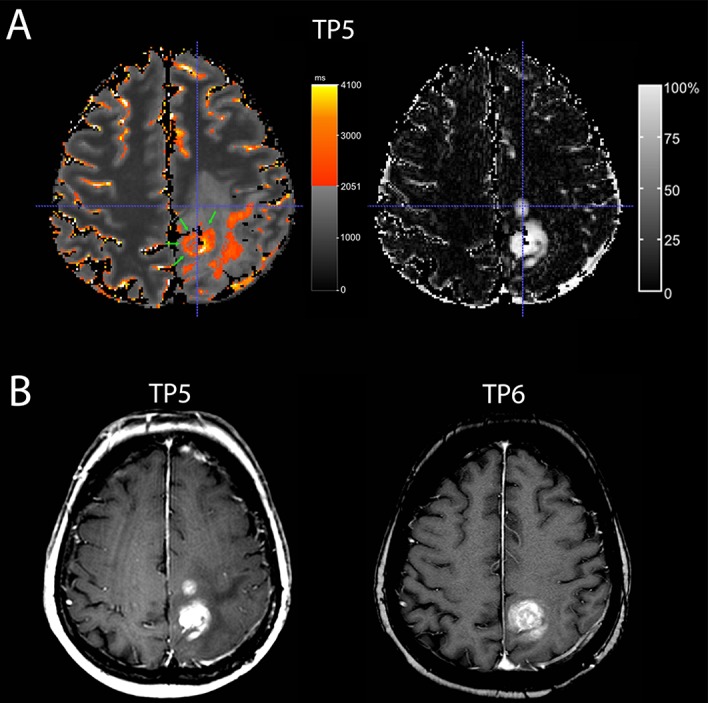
A patient with moderate overlap (patient 9) A Quantitative map with a subtraction map qT1 at time-point TP 5) (A) and conventional MRI at TP 5 and TP 6 (B) of a 70-year-old woman with recurrent GBM. At TP 5, the quantitative map (A, on the left-hand side) shows pre-GBCA qT1 area with >2051 ms (red to yellow, green arrows) with a good overlap of the dorsal contrast-enhancing tumor seen on the subtraction map ΔT1 (A, on the right-hand side), but the second smaller contrast-enhancing tumor area (crosshair) is missing on the color map. This contrast-enhancing area without overlap (crosshair) regressed in the next conventional MRI (TP 6) (B), whereas the contrast-enhancing tumor with overlap progressed. Also, note additional area of T1-prolongation outside the contrast-enhancing tumor.
